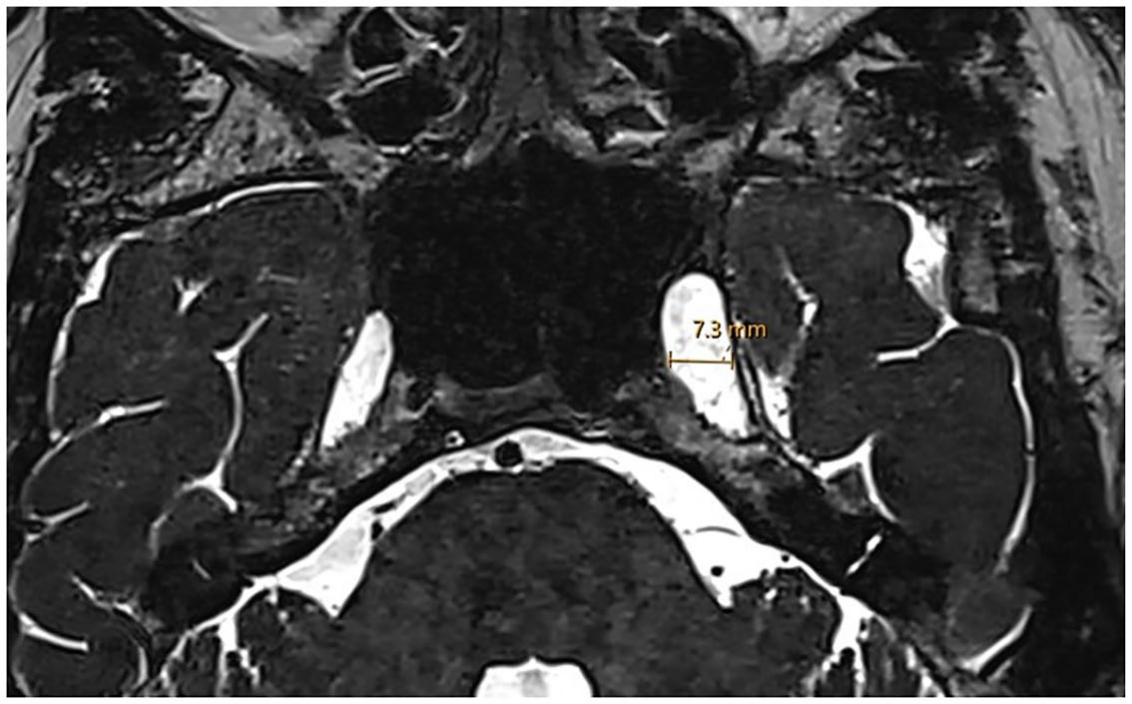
Enlarged Meckel’s cave. Axial 3D T2w SPACE MRI shows an enlarged left Meckel’s cave with a transverse diameter of 7.3 mm.
